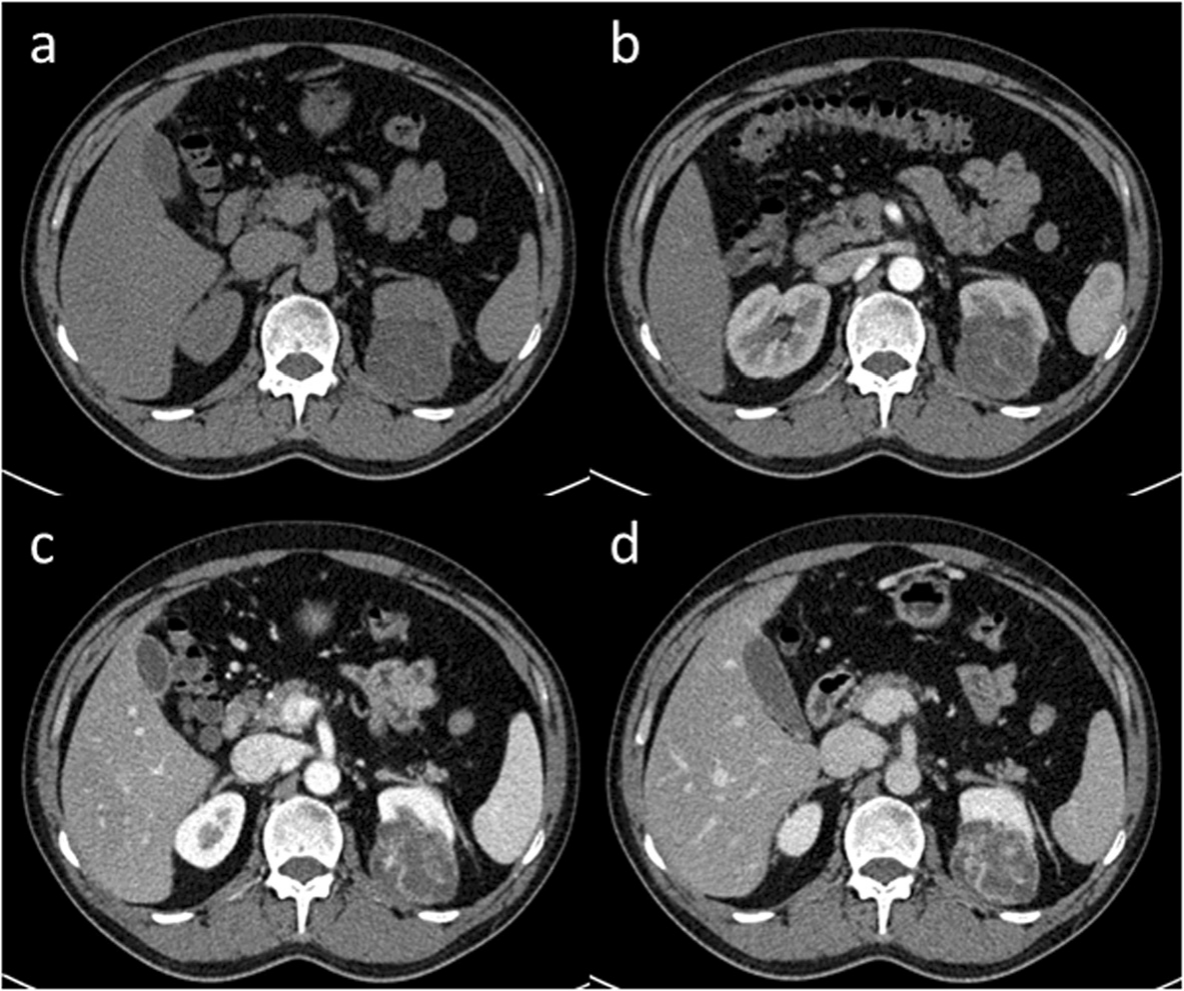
A 41-year-old male with an incidentally discovered left-sided renal mass. Axial non-contrast image (a) demonstrates an exophytic mass with hypo-density arising from the posterior aspect of the left kidney. Contrast-enhanced CT images of arterial (b), venous (c), and delayed-phase (d) demonstrate progressive heterogeneous enhancement with enlarged blood vessels presented in the lesion
